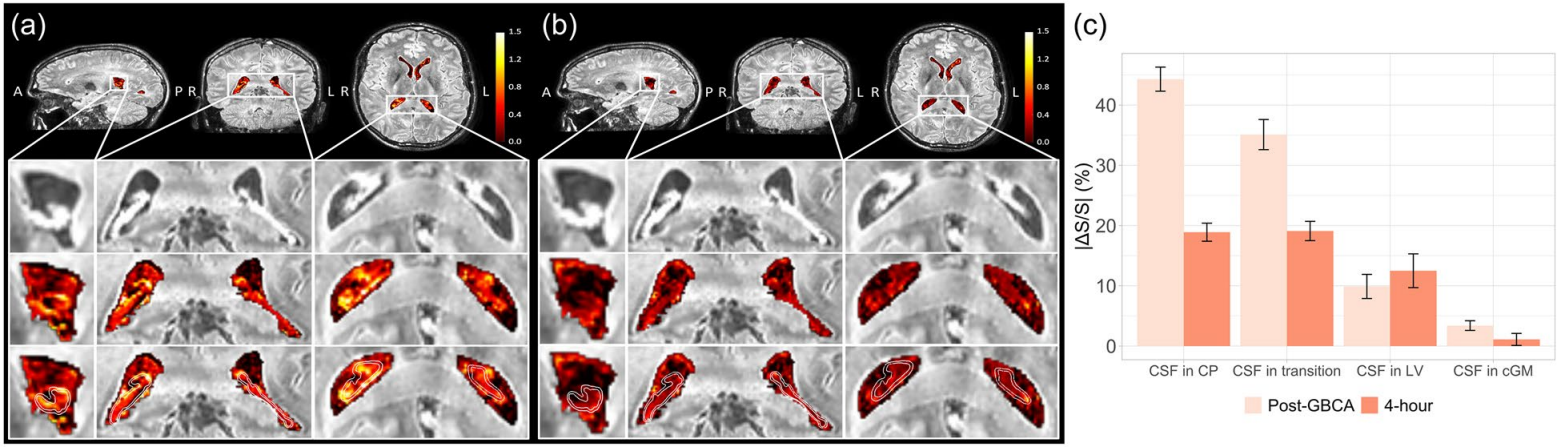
GBCA induced signal changes in the choroid plexus (CP) measured by FLAIR MRI. a Representative maps of relative signal changes (ΔS/S) in the lateral ventricle (including the CP) approximately 6 min after intravenous GBCA administration from a healthy human subject (31 years old, female) are shown. The signal changes were overlaid on the post-GBCA FLAIR MRI images. The scale bar indicates the range of ΔS/S. Note that the FLAIR MRI method has a positive contrast for GBCA-induced signal changes in the CSF. The regions in the white boxes on the first row are magnified on the second to fourth rows. The images on the second to fourth rows are identical anatomically with the CP and transition area between CP and CSF outlined with white contours on the fourth row images. A: anterior; P: posterior; R: right; L: left. b Representative maps of relative signal changes (ΔS/S) in the lateral ventricle (including the CP) approximately 4 h after intravenous GBCA administration from the same healthy human subject are shown. The signal changes were overlaid on the post-GBCA FLAIR MRI images. c Average absolute ΔS/S immediately and 4 h after intravenous GBCA administration measured by FLAIR MRI from all healthy human subjects (n = 25). The signal changes in four ROIs were calculated: the choroid plexus (CP), transition area between CP and CSF (transition), the rest of lateral ventricle (LV), and the perivascular space of occipital cortical grey matter (cGM). The error bars represent inter-subject standard errors. Quantitative results are reported in Table 4. FLAIR: fluid-attenuated inversion recovery; GBCA: gadolinium-based-contrast-agent; CSF: cerebrospinal fluid
